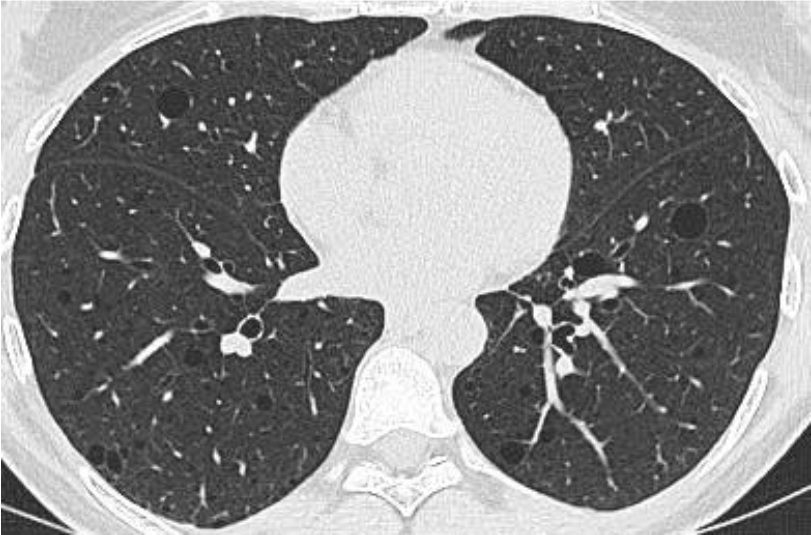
CT scan: multiple cysts in lungs
